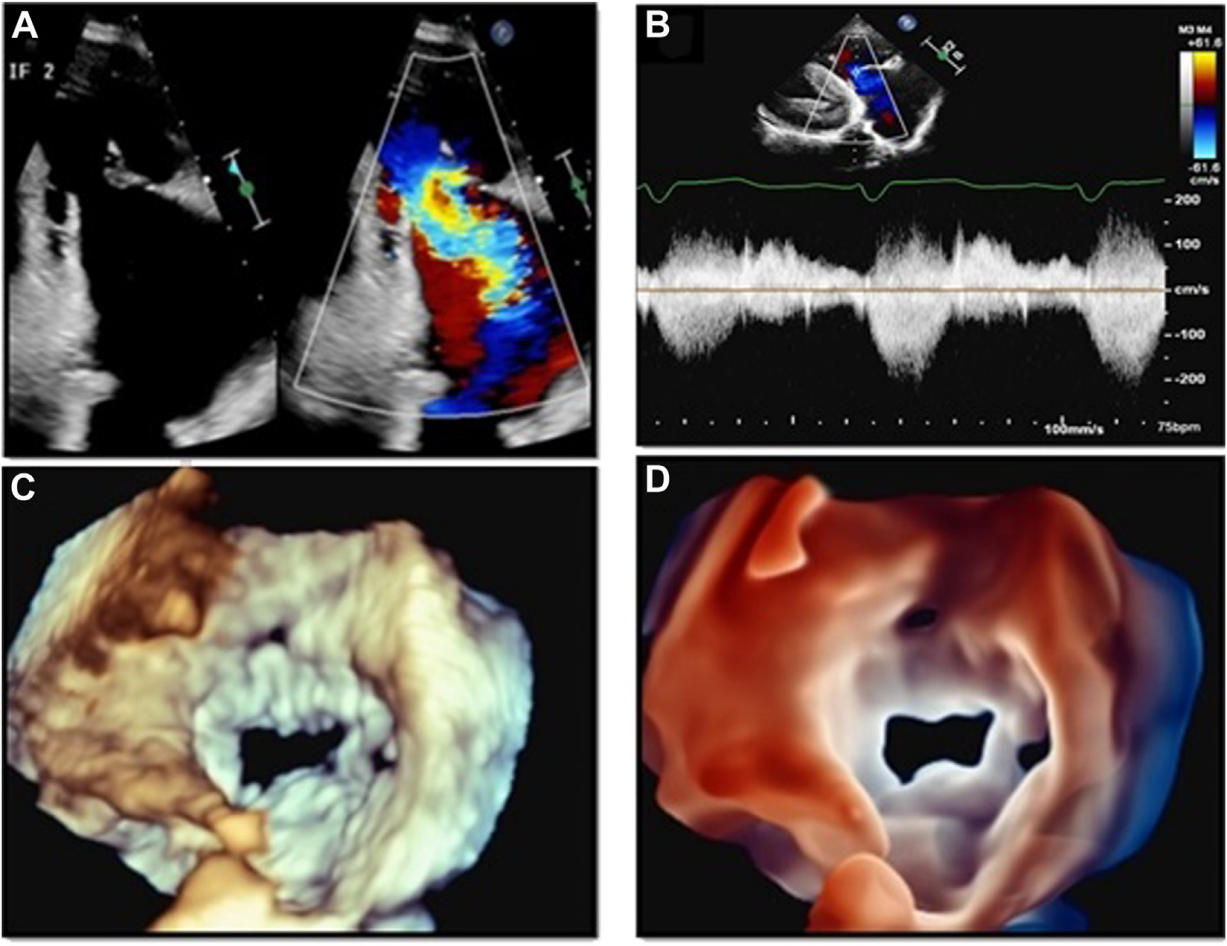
Optimized visualization of TV anatomy with TI. Panel A shows the presence of a midsystolic malcoaptation gap of the TV *(left image)* and massive TR evidenced by color Doppler *(right image)*. Panel B shows a midsystolic triangular-shaped CW Doppler tracing of the TR. Panel C shows a 3D zoom rendering of the TV as seen from the right ventricle, depicting the presence of 4 TV leaflets. Panel D shows a TTE TI rendering of the TV from the RV perspective.
